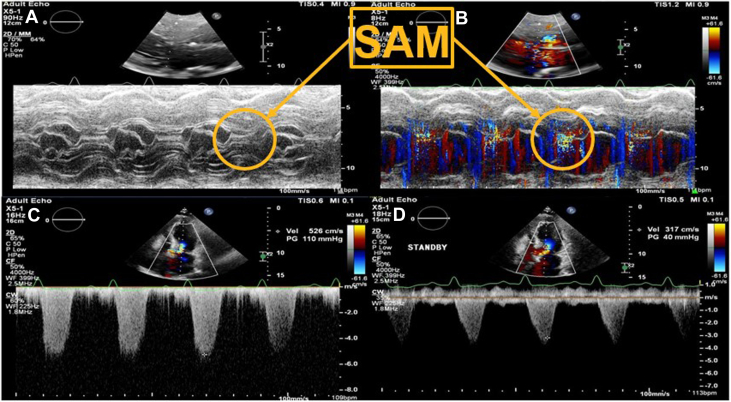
Transthoracic Echocardiogram with IABP at 1:1 Augmentation and High-Dose Vasopressors (A) M-mode showing SAM of mitral valve. (B) Color Doppler M-mode showing SAM of mitral valve. (C) Continuous-wave Doppler showing severe LVOTO. (D) Continuous-wave Doppler showing improvement of LVOTO while IABP on standby. IABP = intra-aortic balloon pump; LVOTO = left ventricular outflow tract obstruction; SAM = systolic anterior motion.
